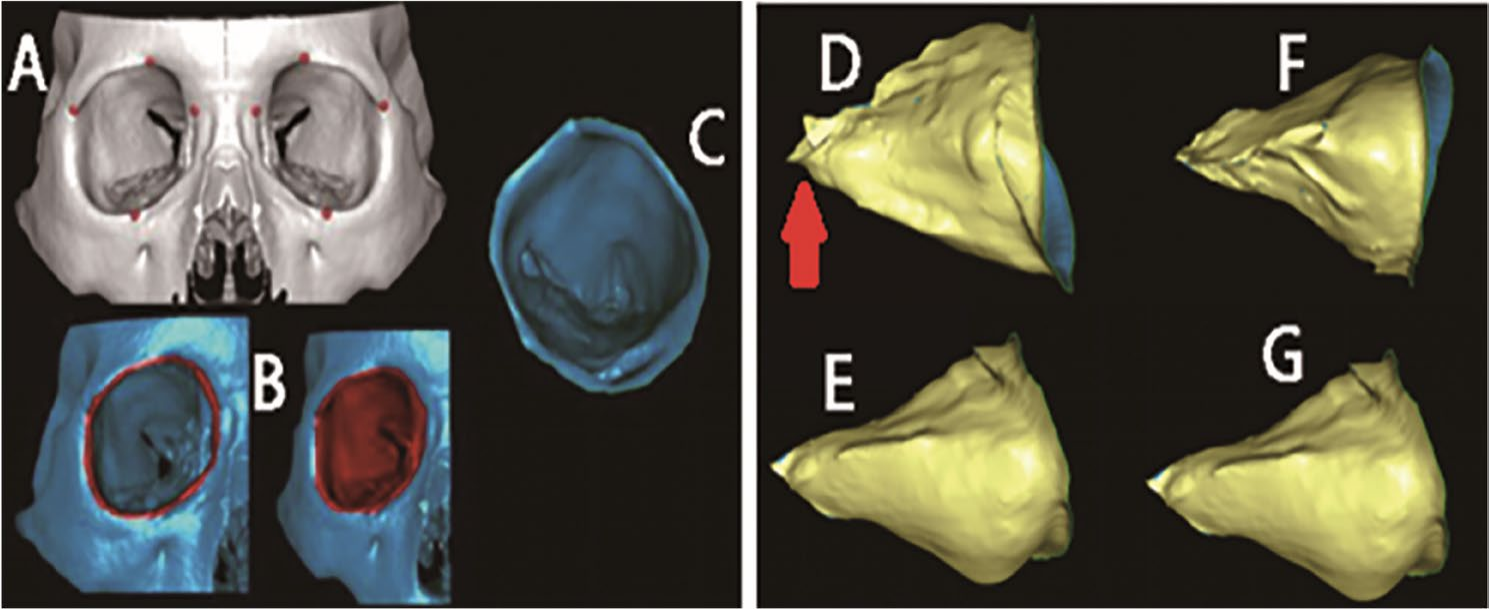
Process of orbital volume measurement for Case 1 using Mango software. (A) Initial placement of annotation points (red crosses) to define the orbital boundaries on an axial CT slice. (B) Results of the semi-automated segmentation: the right panel shows the defined orbital boundary (red outline), and the left panel displays the fully segmented orbital volume (blue overlay). (C) Three-dimensional reconstruction of the segmented right orbit, demonstrating the volumetric ROI(region of interest) used for calculation. (D-G) Multi-angle visualization of the 3D orbital structure: (D) right lateral view, (E) left lateral view, (F) view rotated 90 degrees horizontally from D, and (G) view rotated 30 degrees vertically from E. The orbital cavity is marked in yellow on the outer side, and the optic canal is labeled with a red arrow
